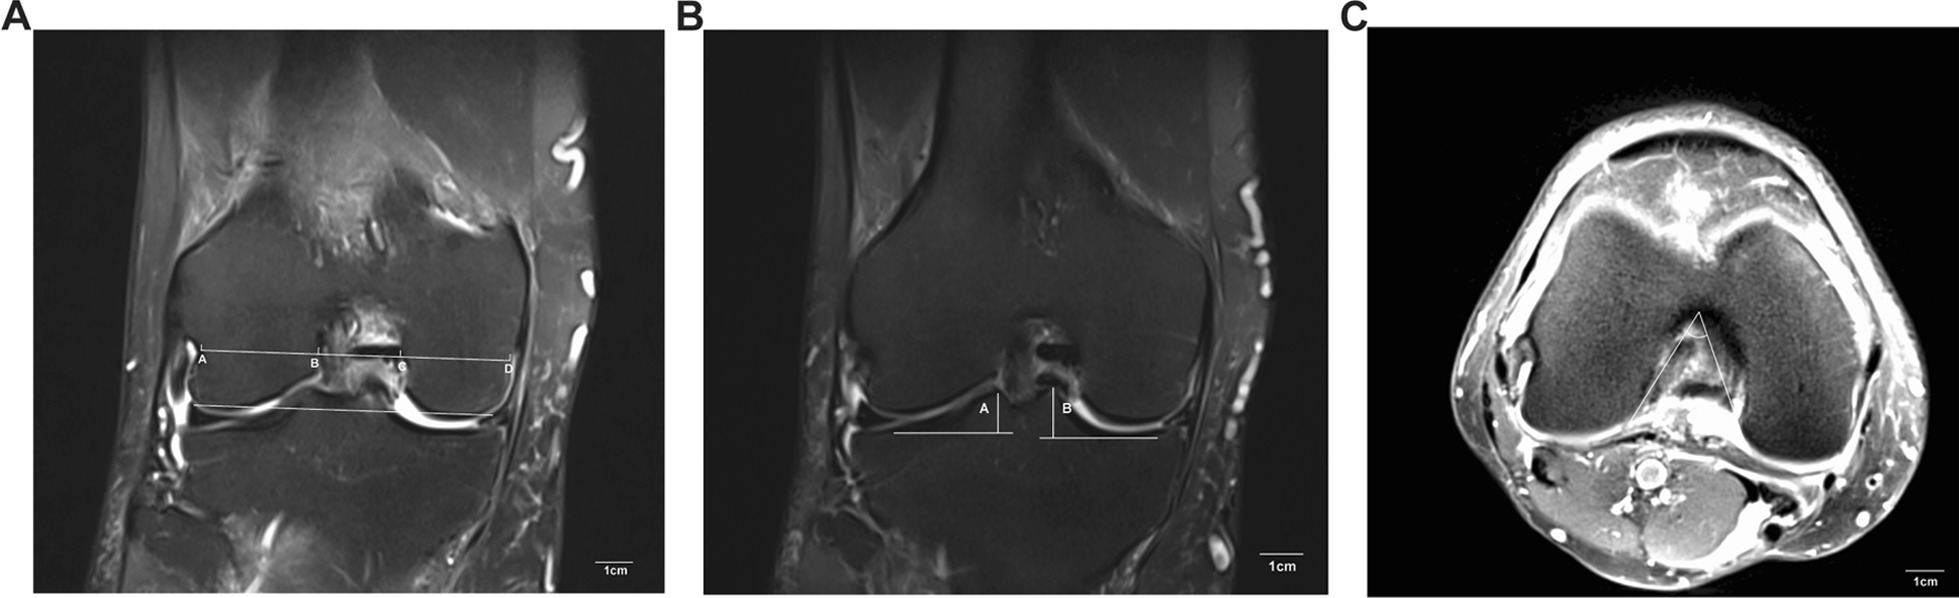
A The measurement of NW, BCW, and condyle width on T2 Coronal view of MRI. A line was drawn through the most inferior boarder of the femoral condyles, and a parallel line was sketched at the level of the popliteal groove. The distance between AB and CD indicated the lateral and medial femoral condyle width, respectively. The distance between AD denoted as the BCW. The distance between BC represented as the NW. B The measurement of the height of medial spine on T2 Coronal view of MRI. The height was defined as the distance from the peak of the tibial spine to the point of the most concave in the tibia. C The intercondylar angle was measured in proton density-weighted axial magnetic resonance images. The angle was shaped by two lines which are from the top of the intercondylar notch to the most inferior border of the intercondylar notch at both medial and lateral condyles
